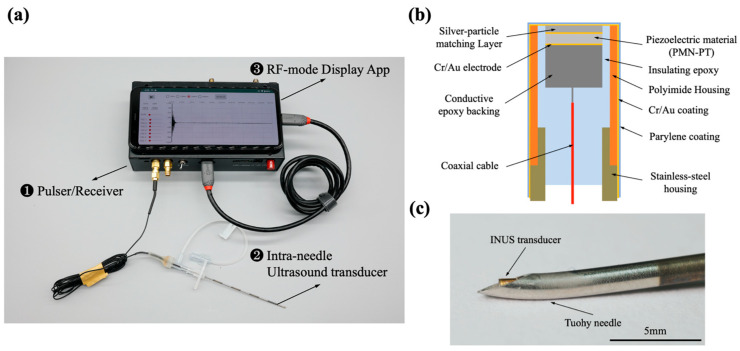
(a) Intra-needle ultrasound system, (b) the design cross-section of the needle ultrasound, (c) the needle transducer fit inside the Tuohy needle.
